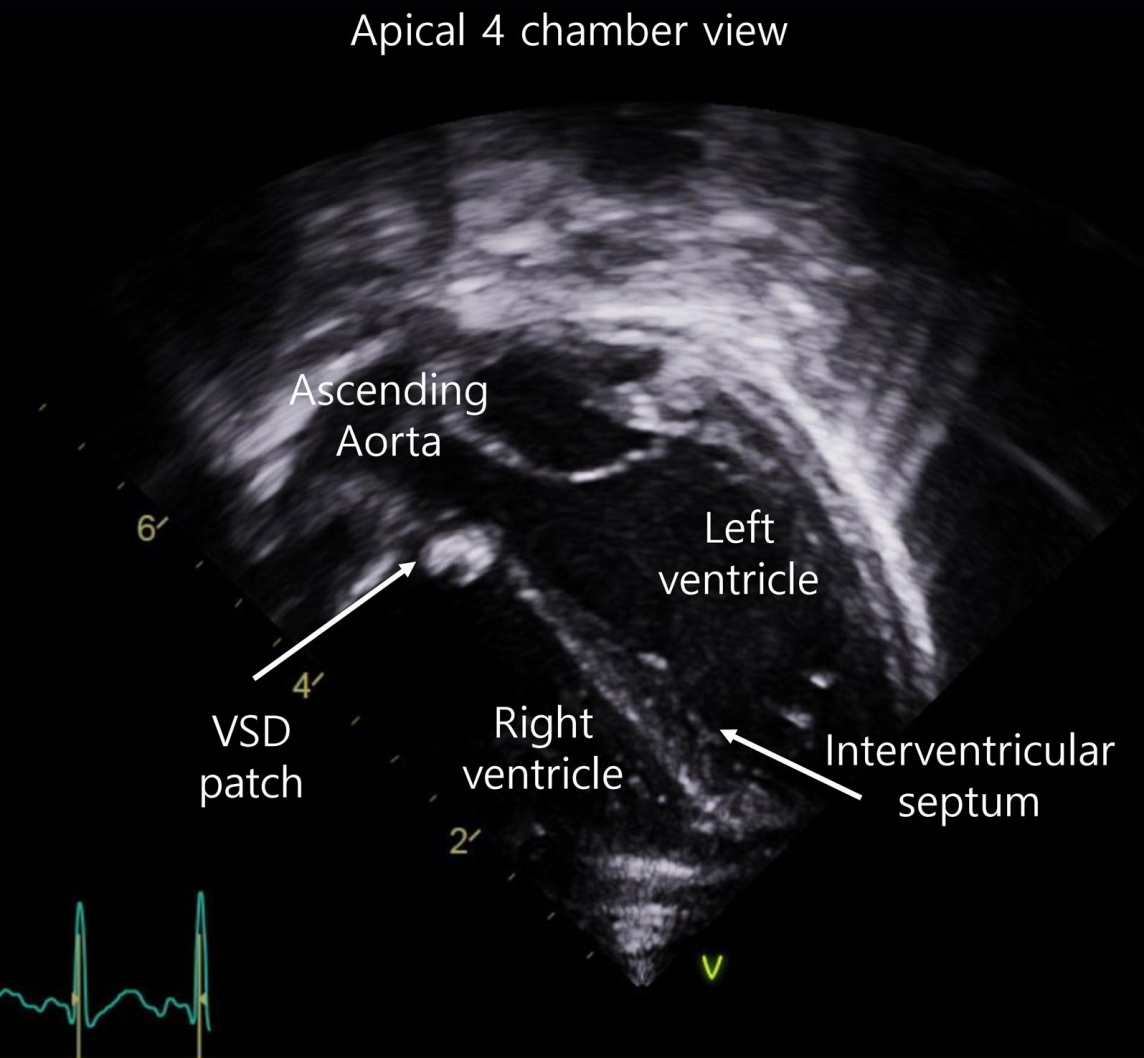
Follow-up transthoracic echocardiography showing a complete resolution of the interventricular septal hematomas in the apical 4-chamber view on postoperative day 40. (Additional file 4: Video S4)
